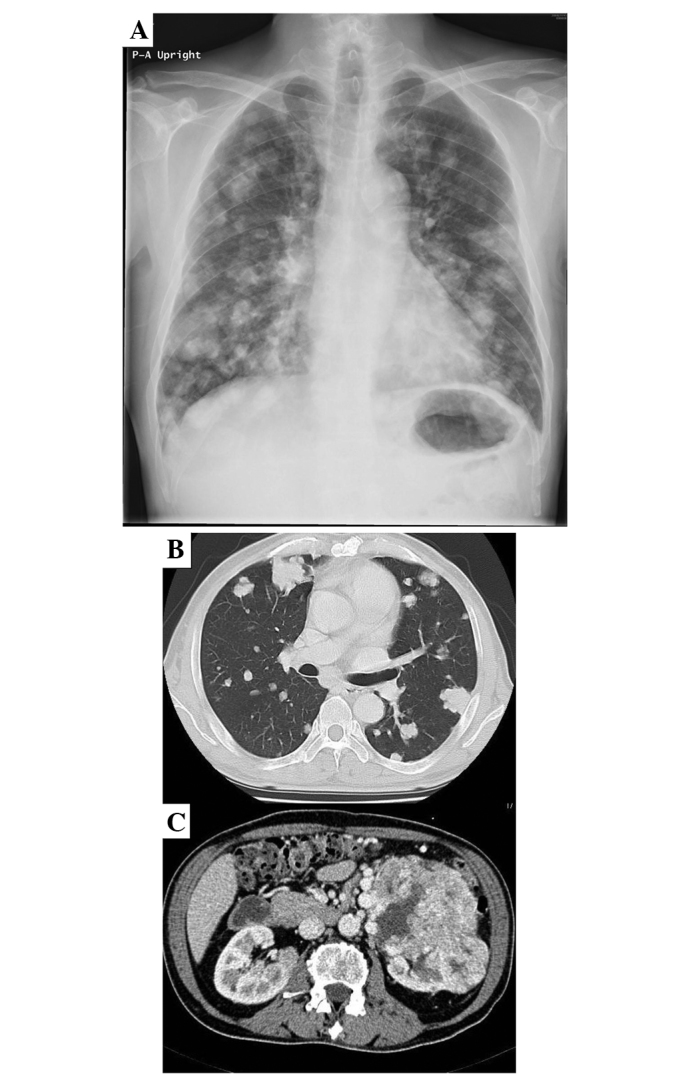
(A) Plain film demonstrating multiple metastatic lesions of the bilateral lungs. (B) Lung computed tomography (CT) also showing multiple metastatic lesions. (C) Screening of abdominal CT revealing left renal cell carcinoma.
